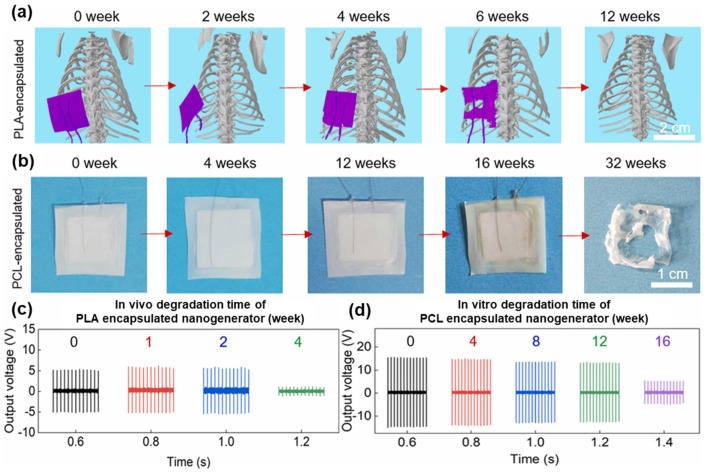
The biocompatibility and biodegradability of PHBV/PLLA/KNN PENG: (a) micro-CT images of the PLA-encapsulated PHBV/PLLA/KNN PENG. (b) Biodegradation performance of the PCL-encapsulated PHBV/PLLA/KNN PENG at 32 weeks. Voltage outputs of the (c) PLA- and (d) PCL-encapsulated PENG measured at different times (ultrasound frequency 100 kHz, ultrasonic intensity 0.3 W/cm^2^). Reprinted with permission from ref. [101]. Copyright 2022, ELSEVIER.
